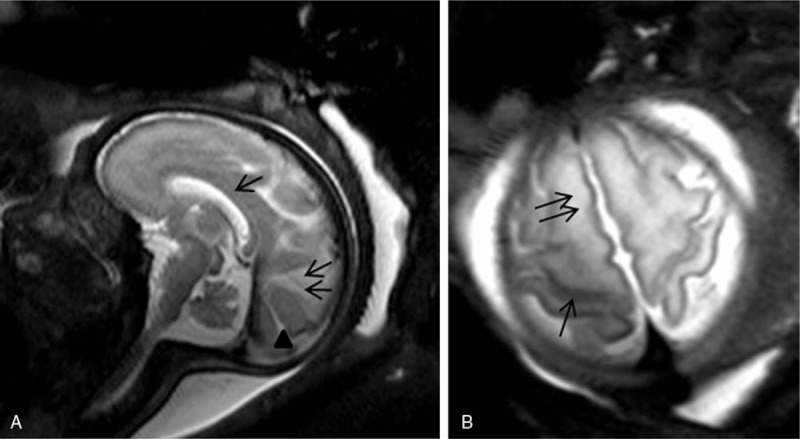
Magnetic resonance imaging (MRI) of the fetal brain at 32 weeks gestation. Sagittal T2 turbo-spin echo (TSE) single-shot image shows the corpus callosum (arrow), the parieto-occipital (double arrows) and calcarine (arrowhead) sulcus [A, Axial T2 TSE single shot image discloses the central sulcus (arrow); B, the superior frontal gyrus (double arrows)].
